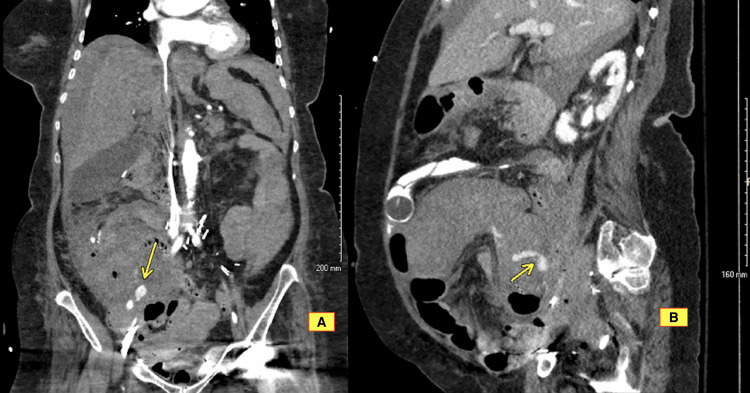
Contrast-enhanced (IV only) CT of the abdomen and pelvis showing postoperative changes with right hemicolectomy and right lower quadrant anastomosis with active contrast extravasation (arrow) at the anastomotic site compatible with active bleeding. (A) Coronal view. (B) Sagittal view.
